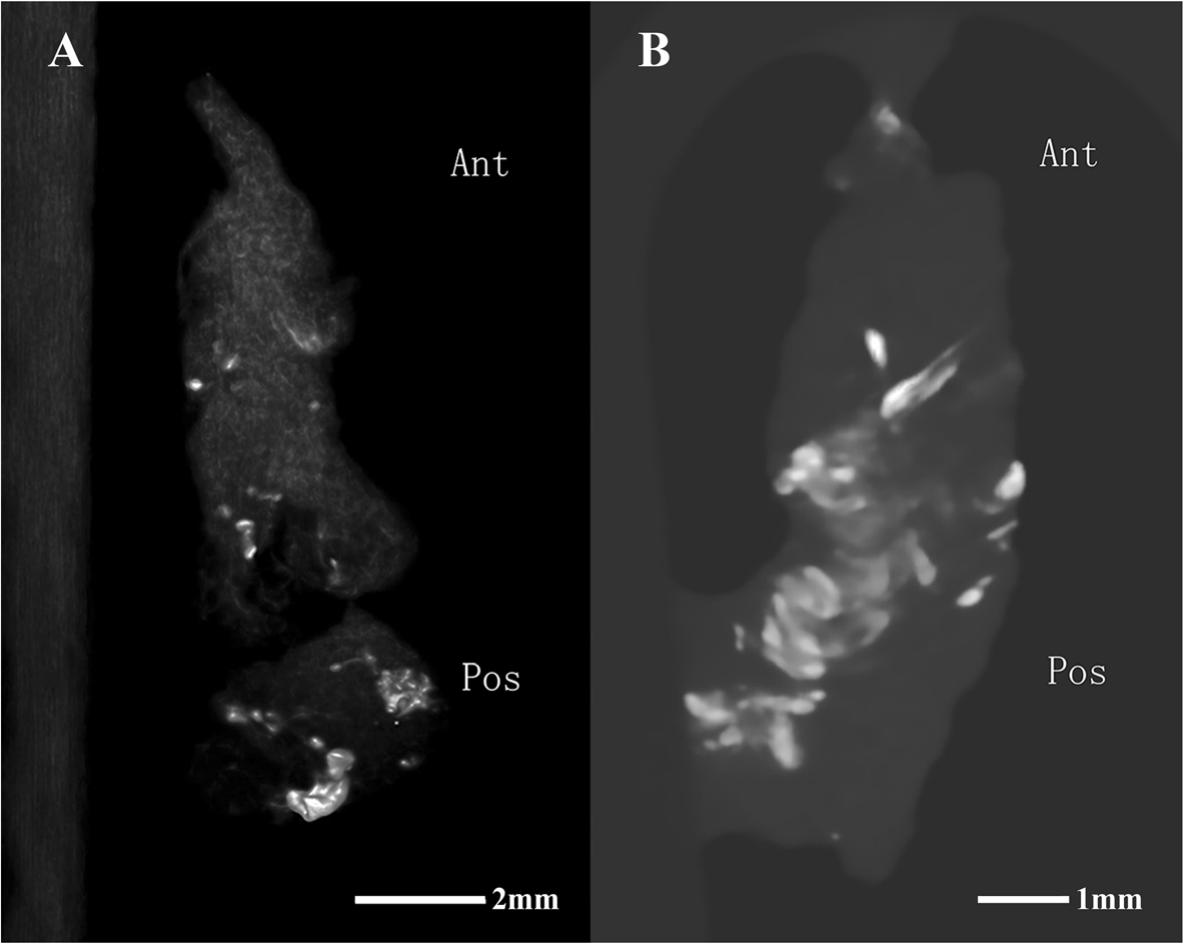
μ-CT scan revealing that the posterior portions of the removed intervertebral discs contained more ossification sites than the anterior portions. White spots indicate the calcification spots in the removed disc. a is a Pfirrmann grade III disc, and (b) is a Pfirrmann grade V disc
